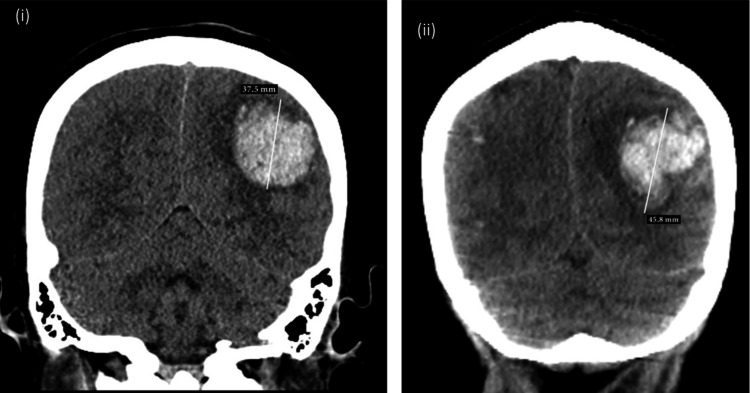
Pre and post-transplant imaging (i) Pre-transplant: CT brain shows intraparenchymal hemorrhage in the left posterior parietal lobe, measuring 3.7 x 2.4 cm, with a mass effect and rightward midline shift of approximately 4.3 mm. (ii) Post-transplant (Day 0): CT brain shows an increase in the size of the largest hemorrhage in the left parietal lobe, now measuring 5 x 3 x 4.5 cm, with increased localized vasogenic edema and a midline shift of 8 mm.
